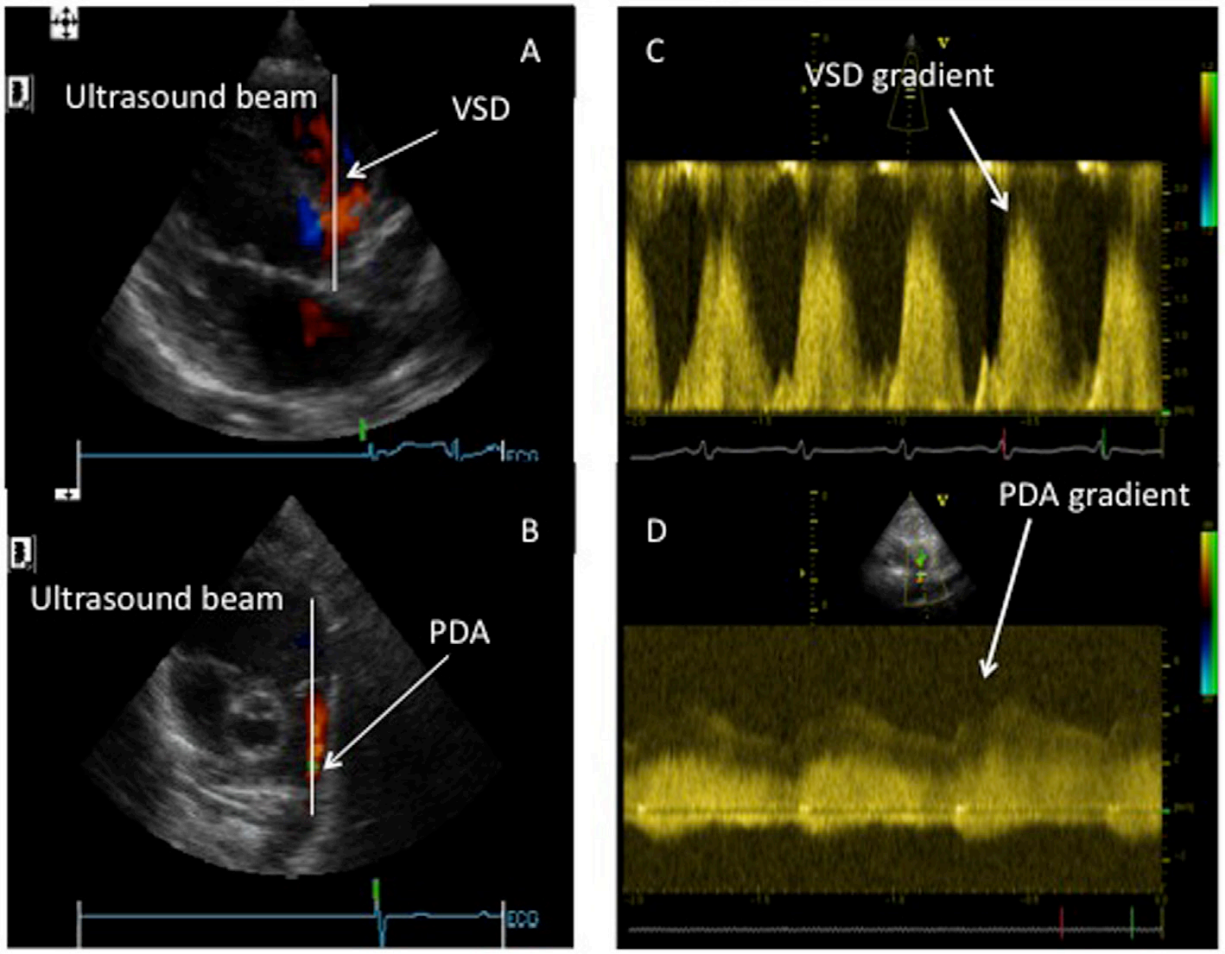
Assessment of systolic pulmonary pressure through the Doppler assessment of post-tricuspid shunts. (A) Doppler beam through a ventricular septal defect (VSD). (B) Doppler bean through a patent ductus arteriosus (PDA). (C) Doppler envelope of the VSD flow allowing for the measurement of the pressure gradient between systemic and pulmonary circulation. (D) Doppler envelope of the PDA flow allowing for the measurement of the pressure gradient between the systemic and pulmonary circulation.
